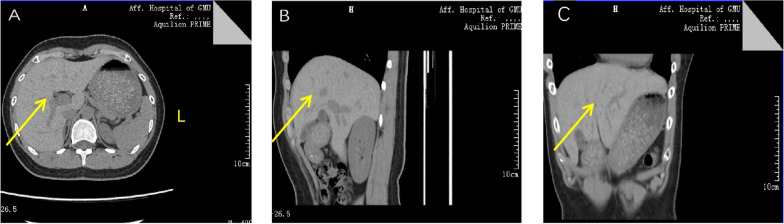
CT images of the upper abdomen. A Horizontal position; B sagittal position; C coronal position. Upper abdomen CT revealed a diffusely increased hepatic parenchyma signal. The liver volume is  slightly enlarged
